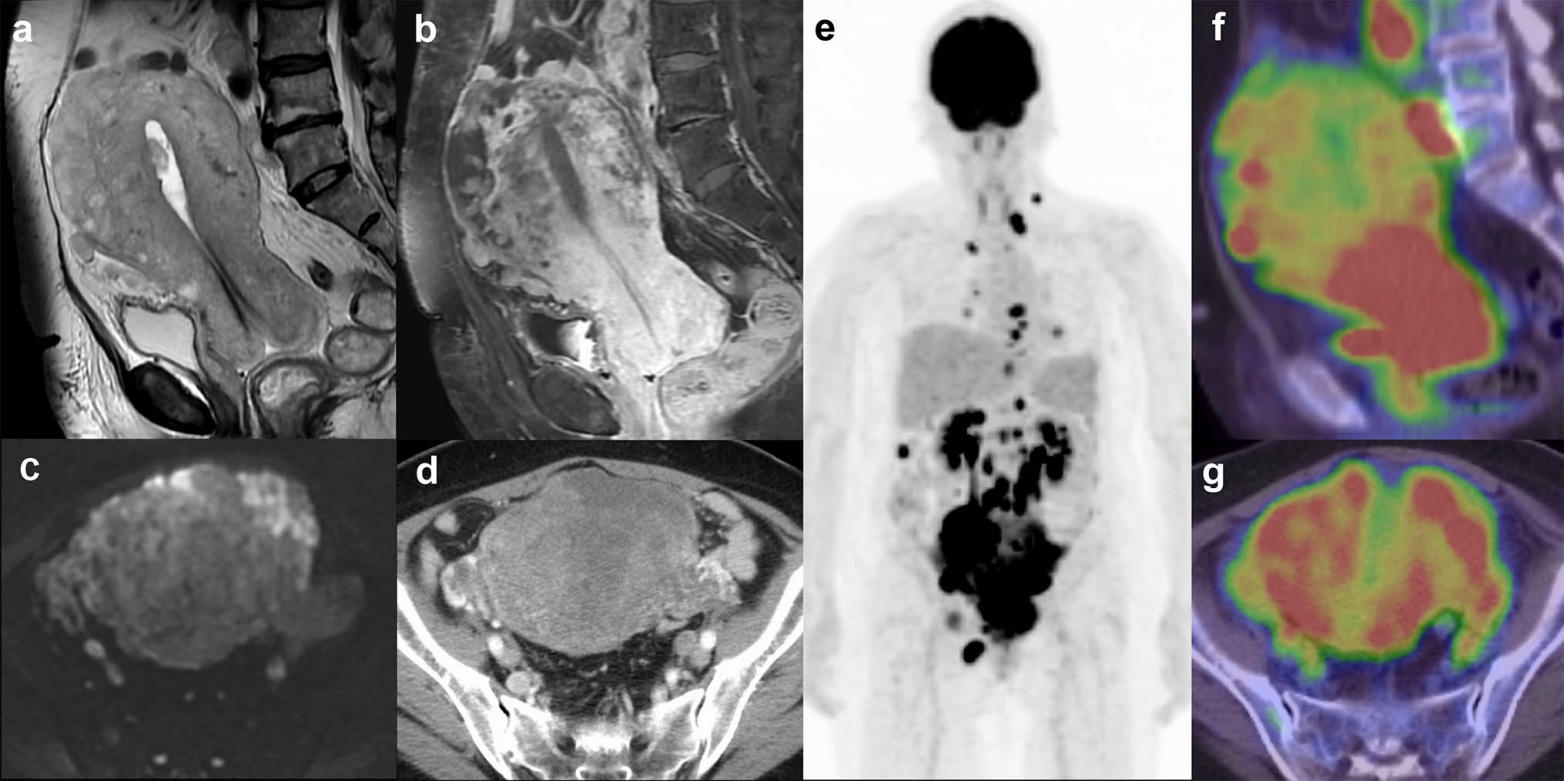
A case of a 61-year-old woman with dedifferentiated endometrial carcinoma (no. 10). a Sagittal T2-weighted image showing an enlarged uterus with an irregular shape and heterogeneous intensity (diffuse involvement pattern). b Sagittal contrast-enhanced T1-weighted image showing heterogeneous enhancement. c Diffusion-weighted (b = 1000 s/mm^2^) image showing diffuse high intensity. Endometrial thickening is not observed. d Axial contrast-enhanced computed tomography showing an enlarged uterus with heterogeneous enhancement. e Maximum intensity projection of positron emission tomography with 2-deoxy-2-[fluorine-18] fluorodeoxyglucose integrated with computed tomography showing a uterine primary carcinoma with multiple metastases from the supraclavicular lymph node to the inguinal lymph node. f Sagittal and (g) axial fused images showing diffuse high 18F-fluorodeoxyglucose uptake in an enlarged uterus. The maximum standardized uptake value of the primary tumor is 14.12
